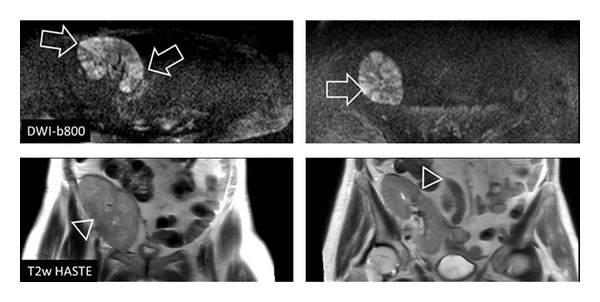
Fifty-eight-year-old female patient with kidney transplant in the right pelvic region. MRI scan showed multifocal, wedge-shaped signal alterations clearly in DWI-b800 (arrow) while T2w imaging could only depict slight pathologic signal (triangle). The diagnosis of nephritis was finally confirmed by urine analysis and laboratory findings.
